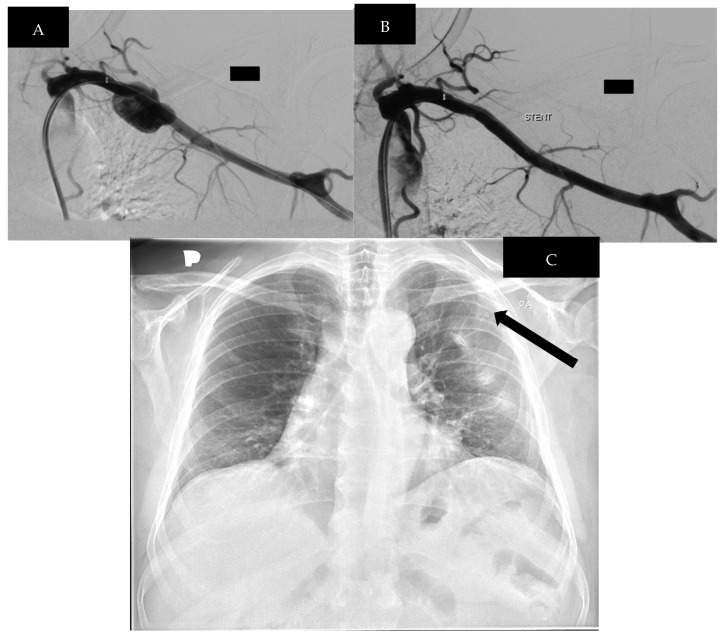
(A,B) Digital subtraction angiography before and after stent implantation. (C) Chest X-ray—showing implanted Viabahn stent. (black arrow).
